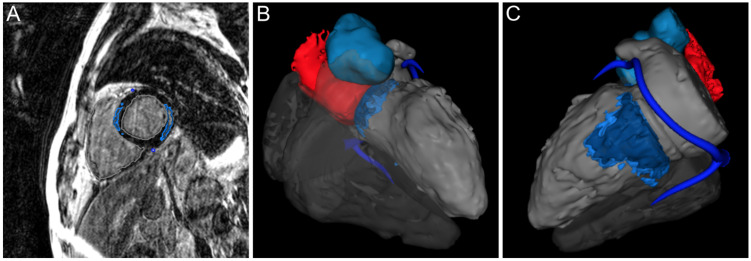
Pre-procedural imaging guides catheter ablation in patients with myocarditis. Exemplary case of a patient with myocarditis who presented with symptomatic premature ventricular complexes (inferior axis, positive in lead I) and non-sustained ventricular tachycardia (CL 258 ms). The patient underwent CMR for 3D imaging acquisition before catheter ablation. (A) Short-axis view of CMR depicting dense scar in a septal and lateral area. (B,C) 3D reconstruction of CMR guides intra-procedural electroanatomic mapping and catheter ablation. Mapping in the right ventricular outflow tract demonstrated earliest activation (42 ms) in the area of septal dense scar and ablation at this localization resulted in complete suppression of premature ventricular complexes and non-sustained ventricular tachycardia. CMR, cardiac magnetic resonance imaging.
